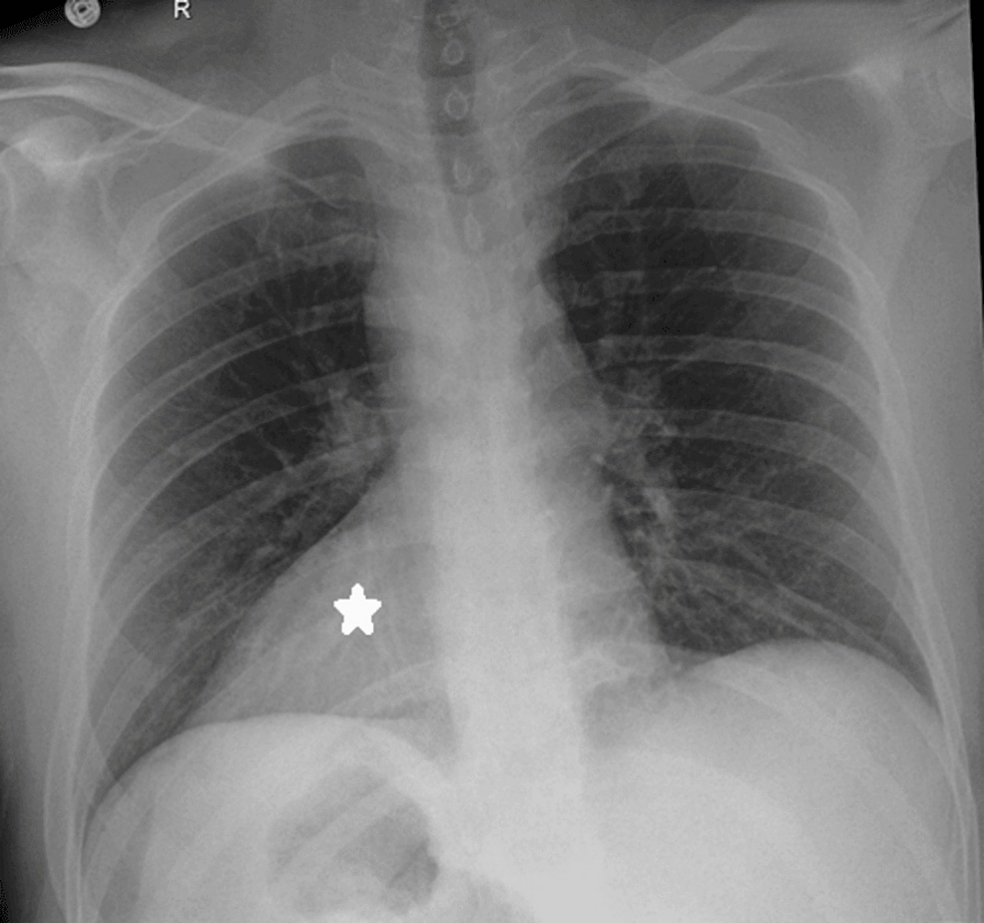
Chest X-ray showing dextrocardia with no signs of pulmonary disease The star denotes the heart in the dextrocardia position.
